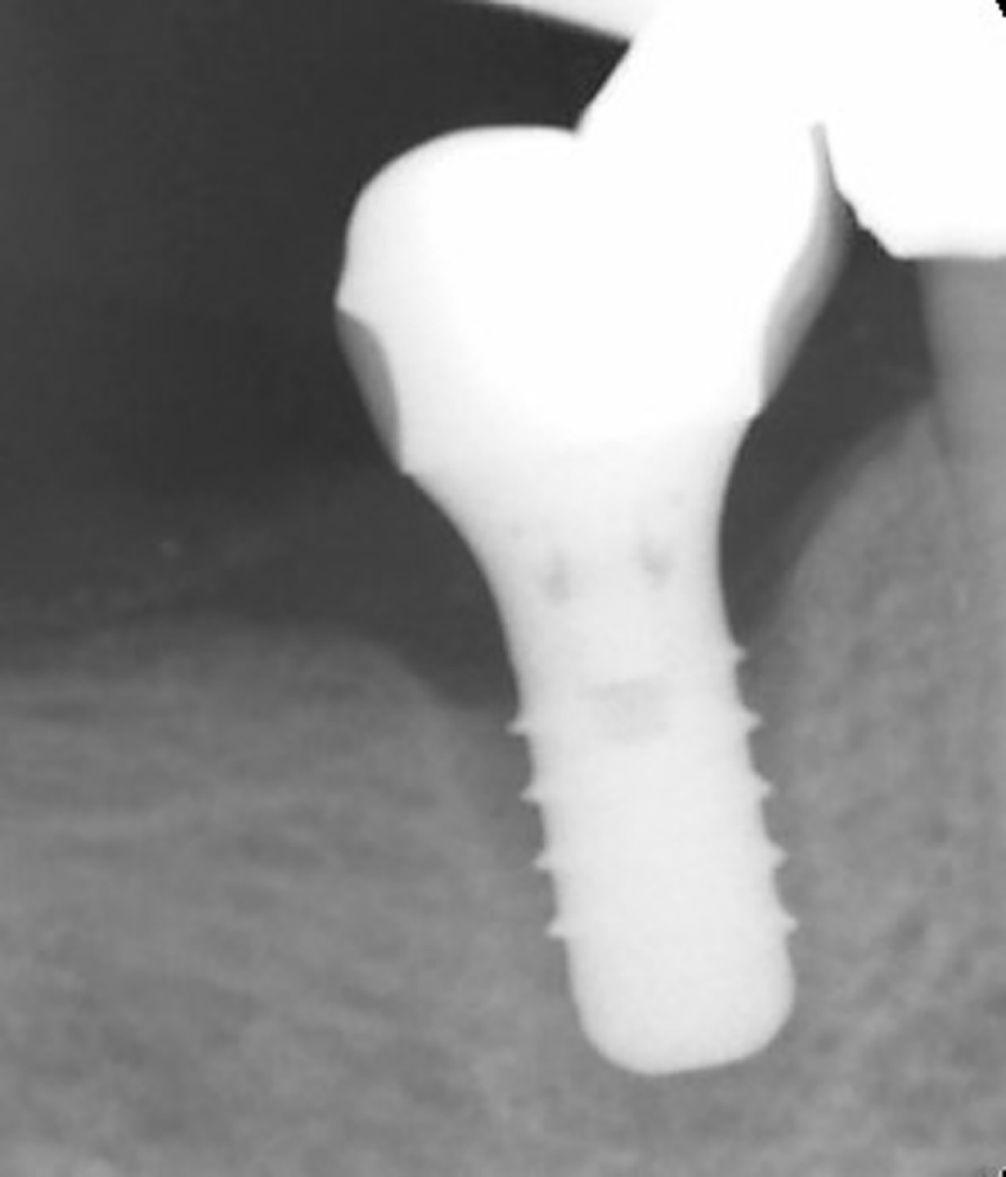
Radiograph that identified a failed implant (3.2 years after implant surgery).
